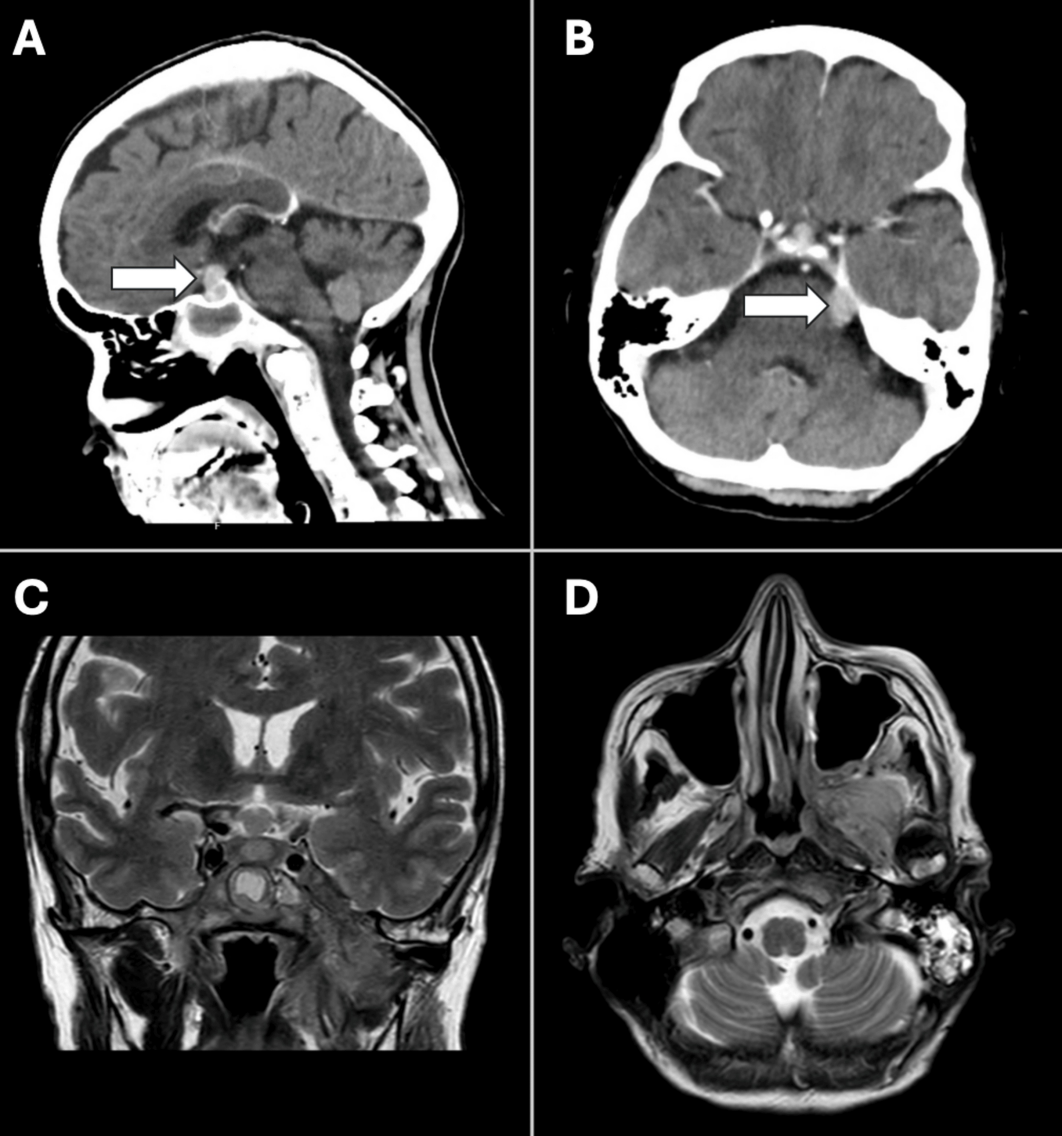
Repeat contrast-enhanced head CT scan six weeks following the initial brain imaging showing a large base of skull tumour filling the sphenoid sinus, extending superiorly to the left orbit. Significant progression in pituitary enlargement is observed, with the tumour extending into the posterior ethmoid sinuses (A). Additionally, there is a new enhancing disease in the left posterior fossa, just below the tentorium, abutting the midbrain but without causing compression (B). MRI pituitary, without contrast, T2-W images show further progression of abnormal soft tissue infiltration into the skull base, masticator space, sella, infundibulum and hypothalamus (C, D).
